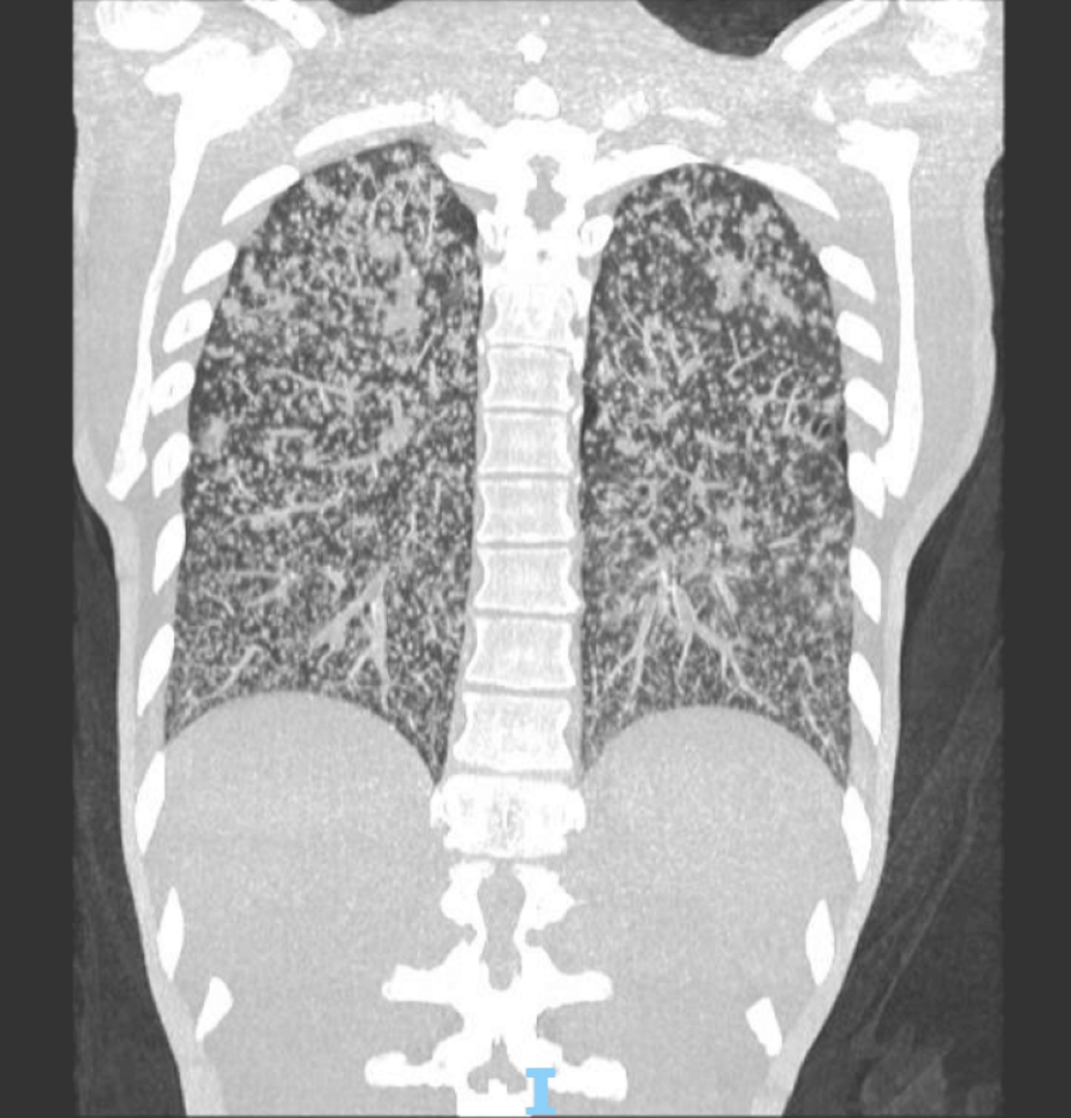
Chest CT scan demonstrating diffuse micronodular infiltrates, mild bilateral peribronchovascular consolidations, and diffuse thickening of the bronchial walls with an infectious-inflammatory pattern, findings consistent with miliary tuberculosis CT: computed tomography
